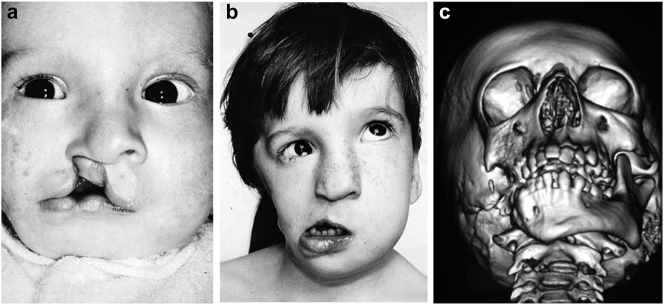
Clinical and CT examples of Class III classification of HFM. (a) Baby with Pruzansky III HFM. (b) Same child 10 yrs later. (c) 3DCT of an example of Pruzansky III HFM.
